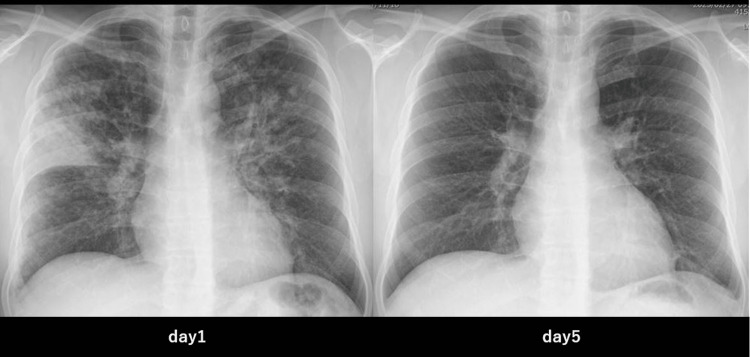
Chest X-ray images at the initial visit and after treatment
